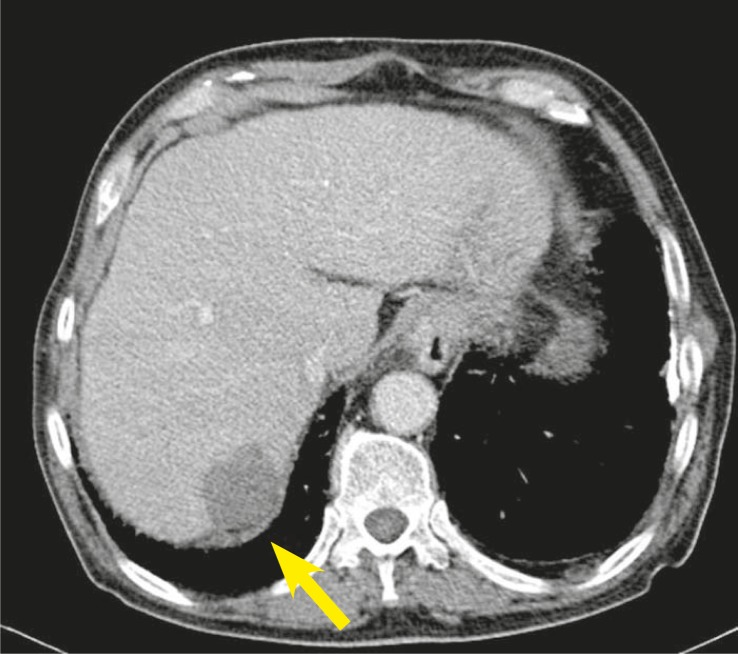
CT scan of hepatocellular carcinoma in right lobe of the liver.
